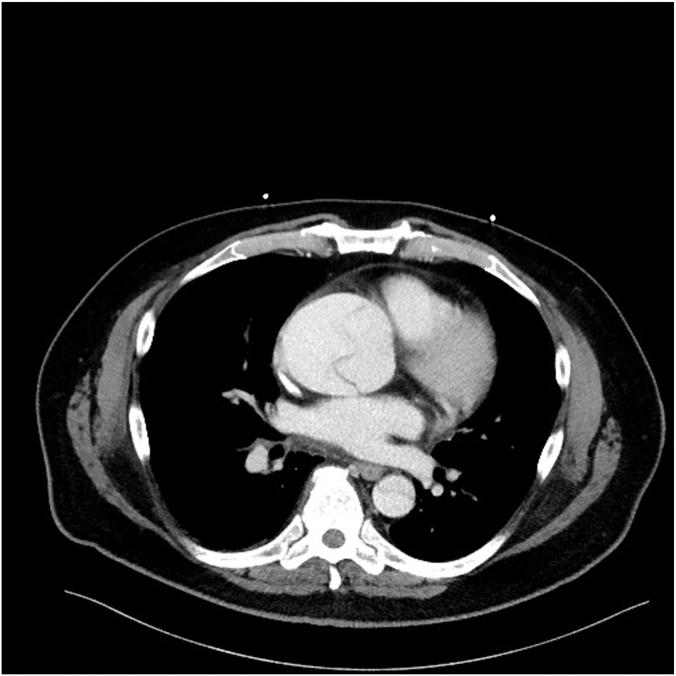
CT angiography of the chestand axial planeshowing dissection of the ascending aorta.
